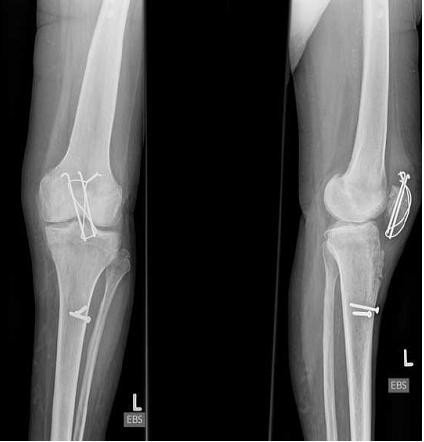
Weight-bearing anteroposterior and lateral radiographs of left knee 6 months after refixation of the tibial tuberosity.
